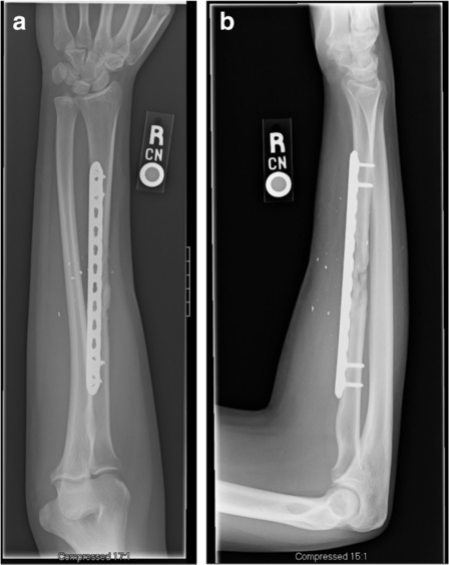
AP (a) and lateral radiographs (b) of the right forearm 4 months post-op.
